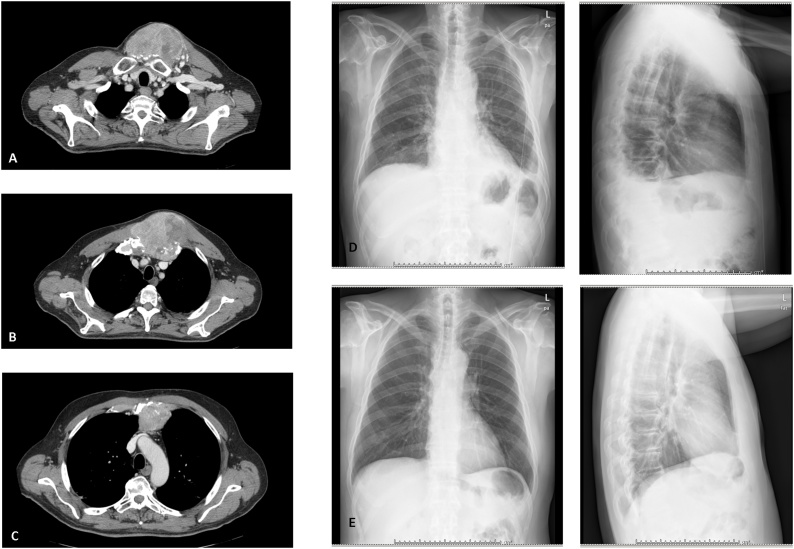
Radiological assessment before and after surgery. A-B-C. Chest CT of the sternal metastasis. Fig. 1B shows a clear infiltration of the manubrium. Fig. 1D. Chest x-ray after 2 days from surgery. Fig. 1E. Chest x-ray after 6 months from surgery.
